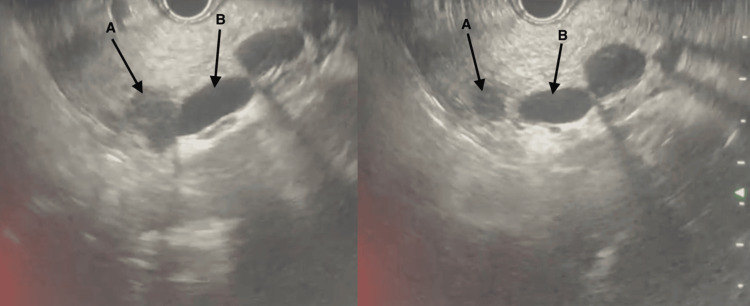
Endoscopic ultrasound The arrow (A) shows a hypoechoic mass at the head of the pancreas. The arrow (B) shows dilated CBD CBD: common bile duct
